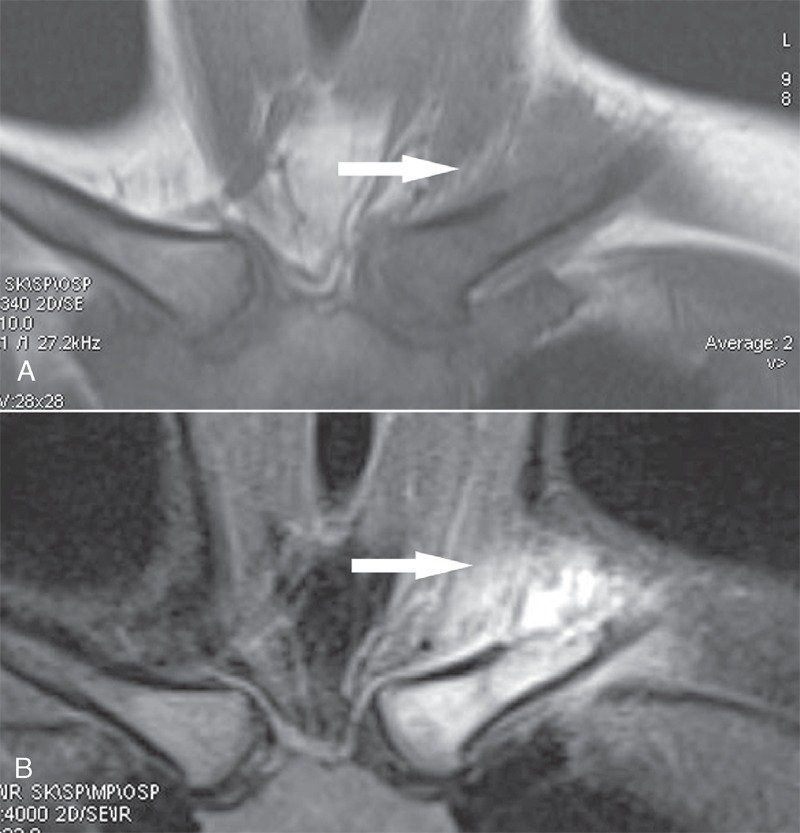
MRI shows the signal intensity of the tumor low in the left clavicle on (A) T1-weighted image and high on (B) T2-weighted image with tumor extension and soft-tissue edema (arrow). MRI = magnetic resonance imaging.
